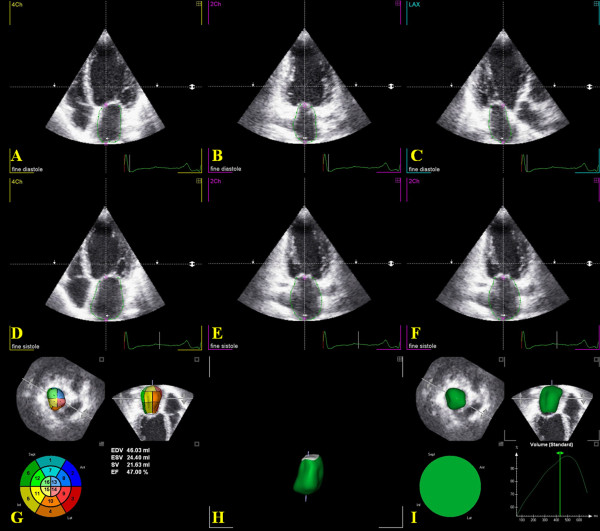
An example of three-dimensional echocardiographic reconstruction of LA volume (ml) and LA ejection fraction (LAEF%) measurements by TomTec software, after the acquisition of a full-volume dataset. Delineation of the endocardial border at end-diastole (A-C) and end-systole (D-F), estimation of LAEF (G), LA volume reconstruction (H) and LA time-volume curves (I).
